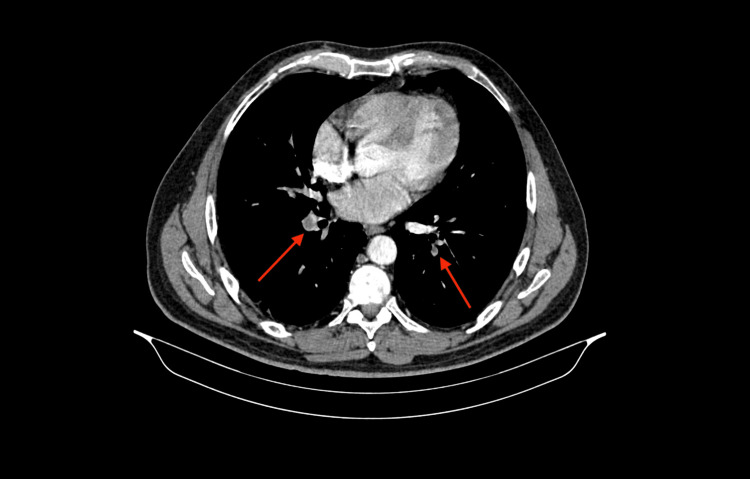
Bilateral pulmonary embolism after the surgery. Hypodense thromboembolic masses (arrows) on the thoracic CT angiogram.
